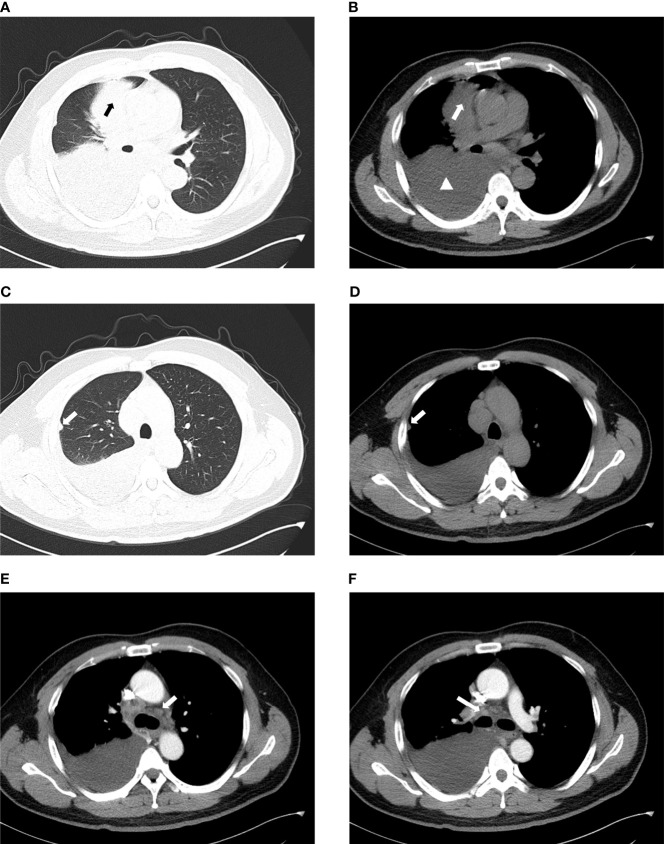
Imaging characteristics of chest CT. (A, B) The mass is located in the right upper lobe and is of central type with moderate to large pleural effusion on the right (arrow: mass, triangle: pleural effusion). (C, D) They show the ipsilateral pleural nodule but no involvement of the contralateral lung (arrow:pleural nodule). (E) Contralateral mediastinal lymphadenopathy. (F) Ipsilateral hilar lymphadenopathy.
